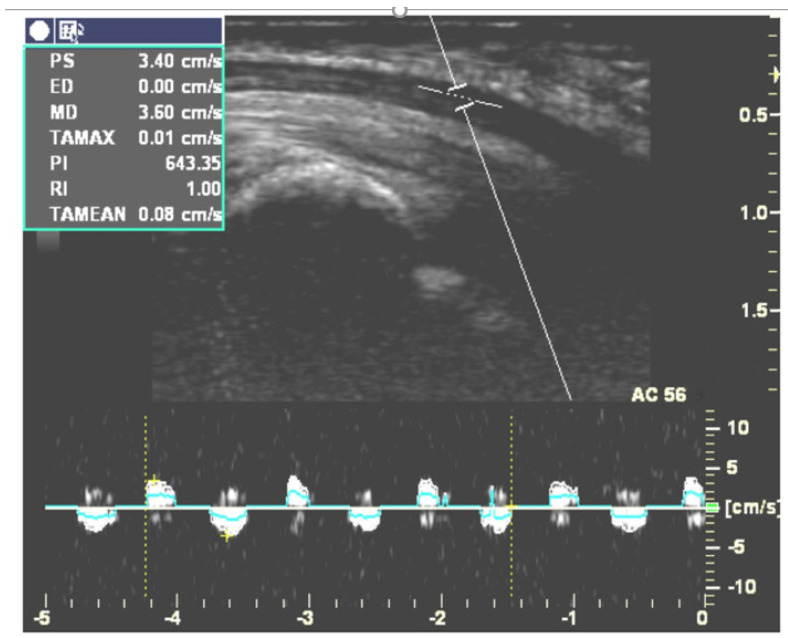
Doppler waveforms for measuring longitudinal MN gliding during active flexion and the extension cycle of the index finger at a speed of one time per second. (This figure was adapted from Liu et al. [33]).
